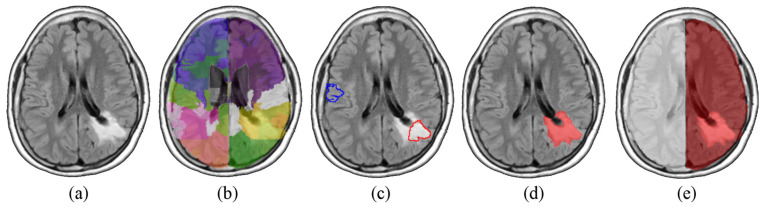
Steps (axial sections) of the procedure for the preliminary tumor mass localization. Each FLAIR image is mapped onto the MNI space (a), and (b) parcelled into ~300 districts (different colors identify different areas). In (c) clear training regions for SVM are selected (typical healthy and affected tissue outlined in blue and red respectively), and in (d) all the areas classified as diseased are merged together in a volume (V_t_, in red). Final decision of the tumor-affected hemisphere (right hemisphere, highlighted in red), by intersecting V_t_ with a coarse mask of the two hemispheres (e).
